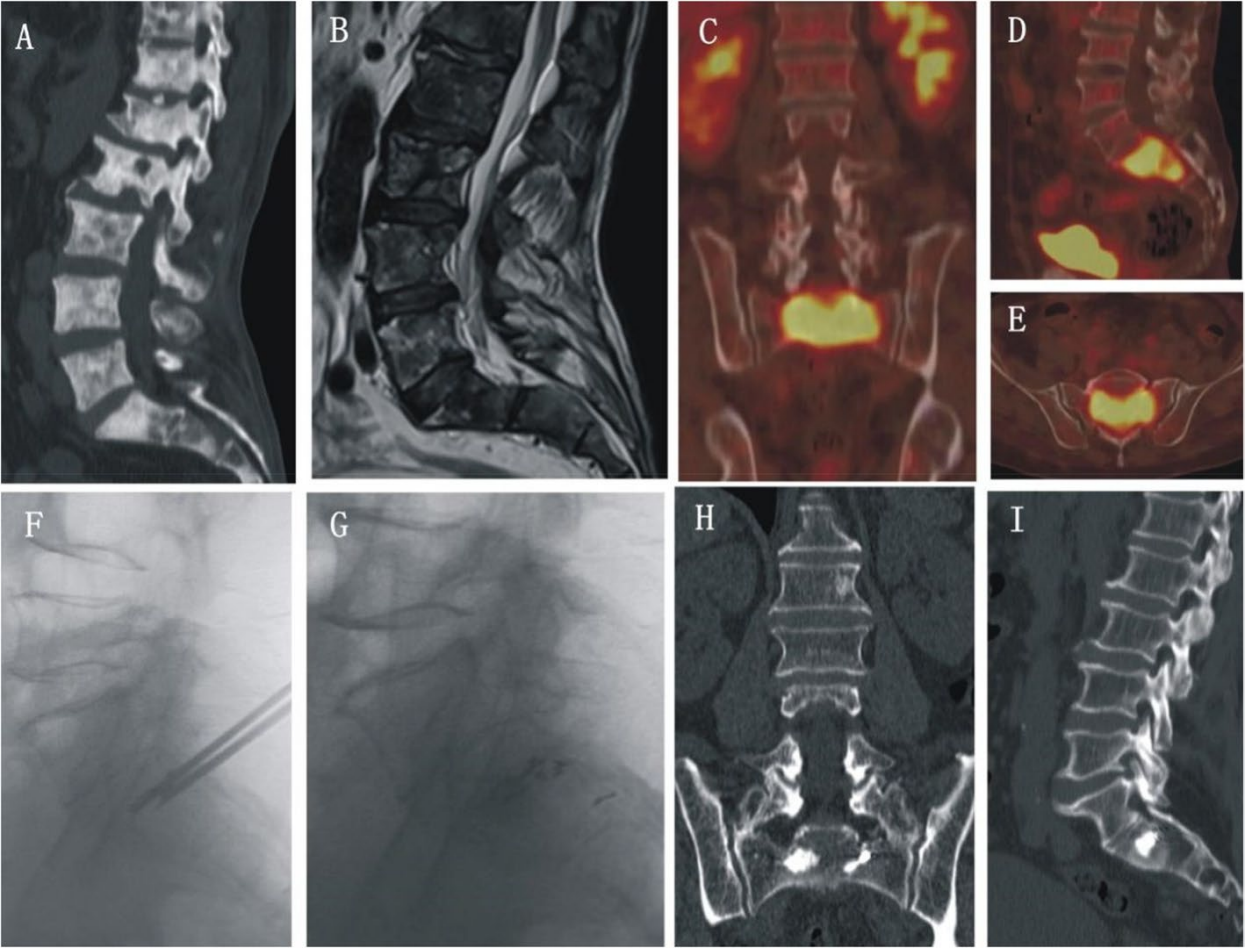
Male patient, 64 years old, with well-differentiated prostate cancer in February after combined treatment, Back pain 2 weeks preoperative VAS score of 8.7. A–E Preoperative CT, MRI, and PET-CT show sacral bone destruction and soft tissue mass formation, sacral lesions involving the sacrum, and the corresponding hole. F–G S2PVP combined with ^125^I seed implantation. H–I Postoperative CT showed bone cement and ^125^I particle distribution in the lesion area, with no leakage of bone cement; Postoperative VAS score, 2.5 points, significant pain relief
